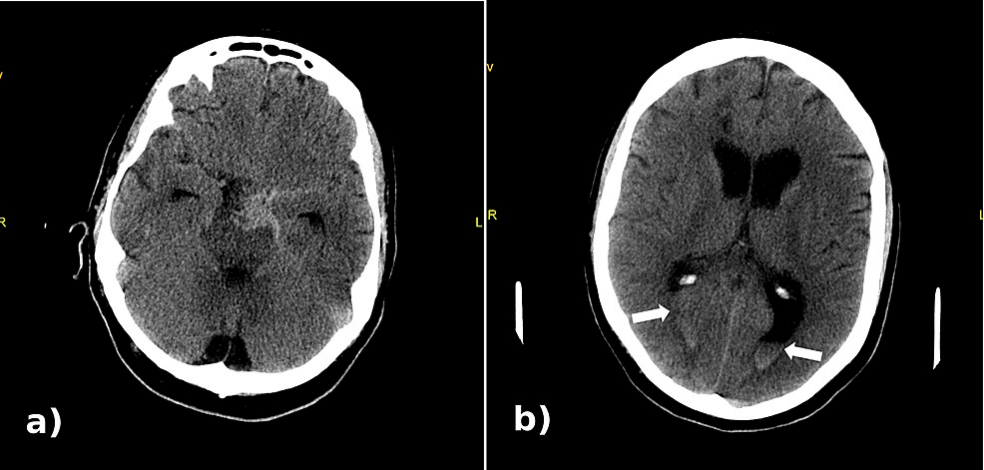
Computed tomography of the head without intravenous contrast (axial plane) a) Computed tomography of the brain shows subarachnoid hemorrhage in ambiens, crural, interpeduncular, and Sylvian cisterns. Note the hypodensity of the midbrain associated with edema. b) Hydrocephalus in lateral ventricles, blood content in the occipital horns of lateral ventricles, and mild hydrocephalous (arrows).
